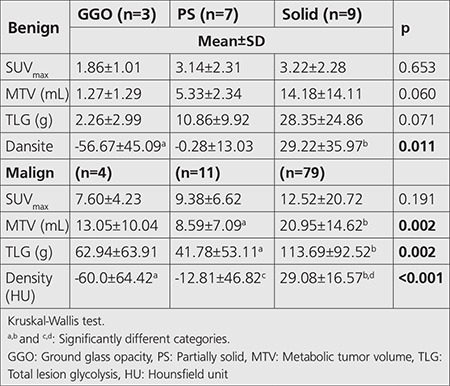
^18^F-FDG positron emission tomography/computed tomography SUV_max_ and volumetric parameters according to attenuation classification
